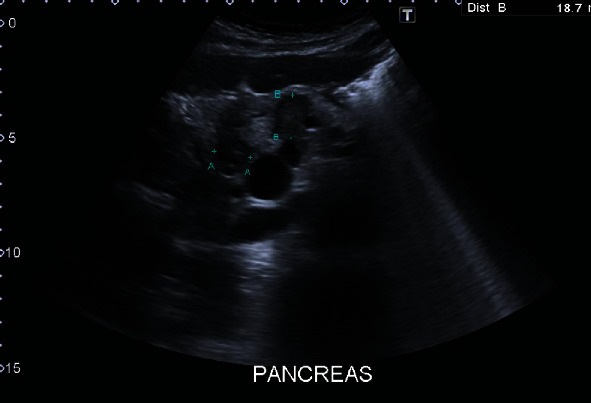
Ultrasound showing hypoechoic masses within the pancreas.
